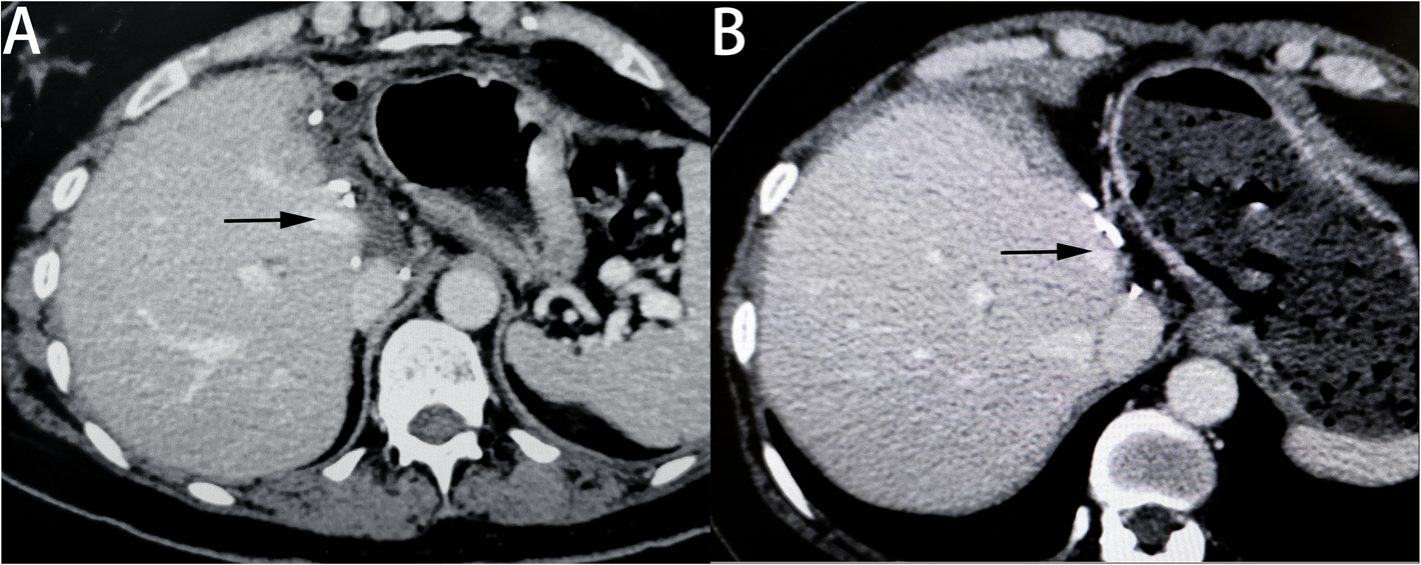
Postoperative enhanced computed tomography. a CT showing the patency of the graft at 1 year after the operation (patient 5). b CT showing the patency of the graft at 2 years after the operation (patient 2)
